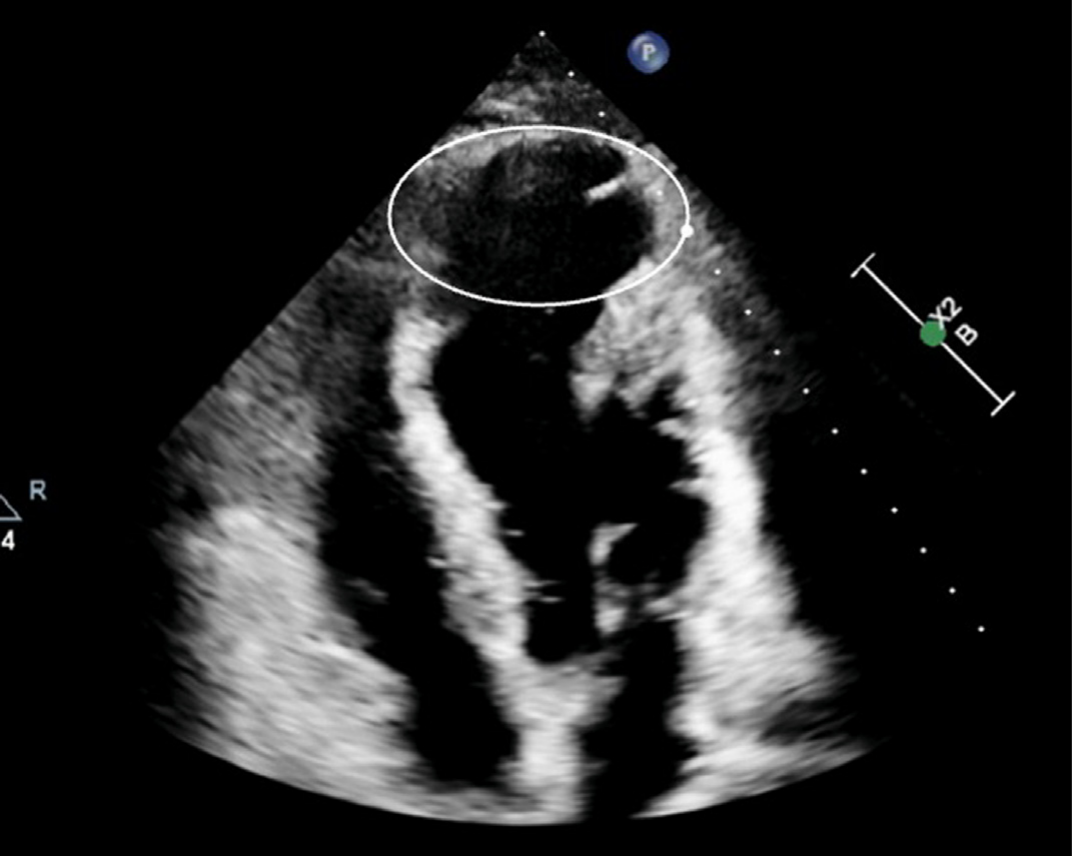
Transthoracic Echocardiogram Axial 4-Chamber View Circle shows prominent papillary muscles and septal protrusions in the left ventricle.
